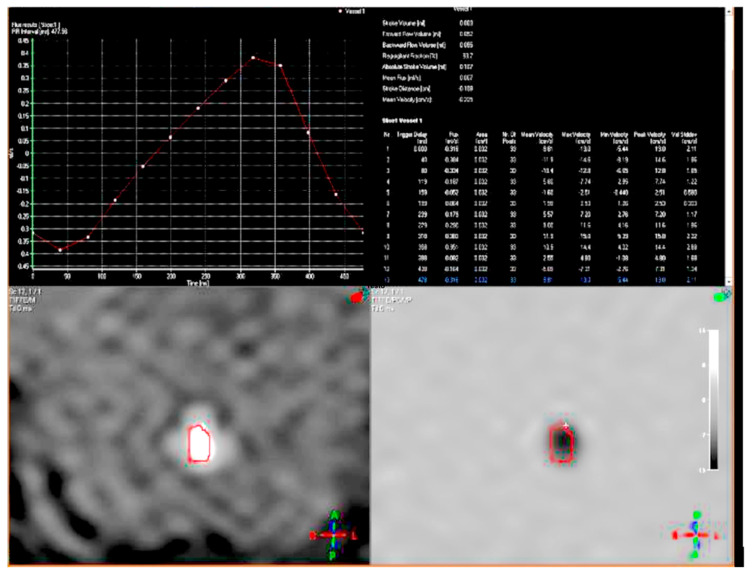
Cine MRI showing a flow turbulence throughout III and IV ventricles.
